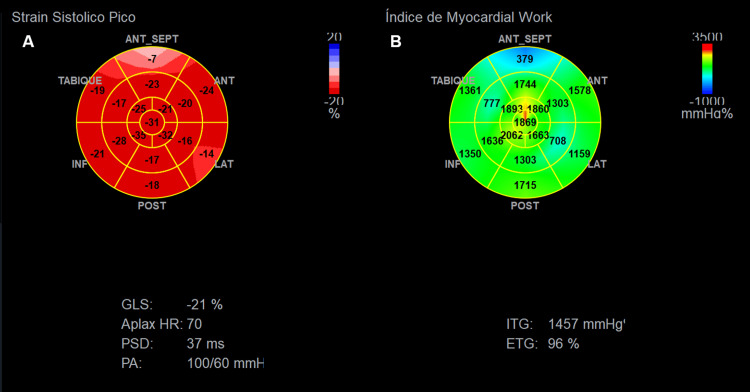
Transthoracic echocardiography (TTE) with global longitudinal strain (GLS) and myocardial work analysis Image A: Bull’s-eye plot of peak systolic strain showing segmental reduction in the basal anteroseptal wall (-7%) and basal-mid anterolateral wall (-14% and -16%, respectively; reference range: >-16%). Image B: Bull’s-eye plot of the myocardial work index demonstrating reduced regional work in basal and mid segments (379-1715 mmHg%), with a global work index of 1457 mmHg% (reference range: >1800 mmHg%). These findings suggest subclinical myocardial dysfunction.
